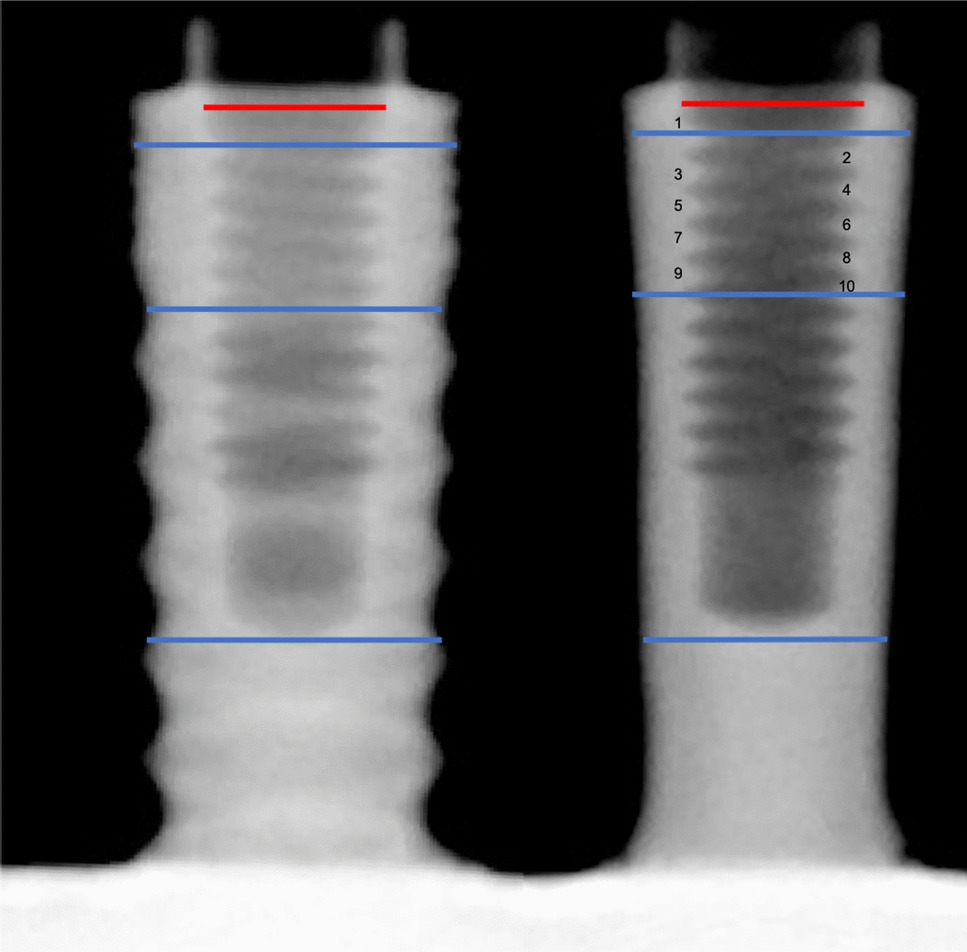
Radiographic measurements of the implant wall width. Left: control implant; right: IP implant. Blue lines: length at middle of the first (R1) and tenth (R2) threads and at the end of the prosthetic screw hole (R3), perpendicularly to the long axis of the implant; red line: 1.9 mm reference
